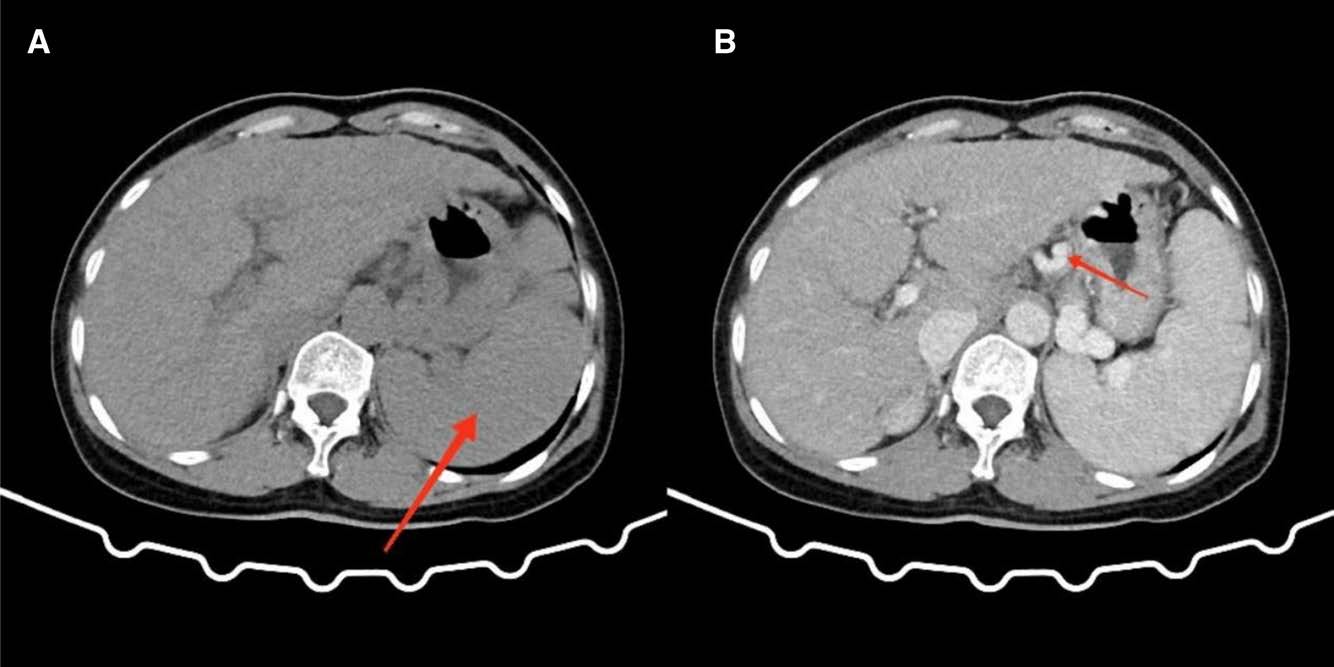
Upper plain and contrast-enhanced abdominal CT scans. (A) Red arrow indicates splenomegaly. (B) The portal venous phase CT, with the red arrow pointing towards gastric varices.
